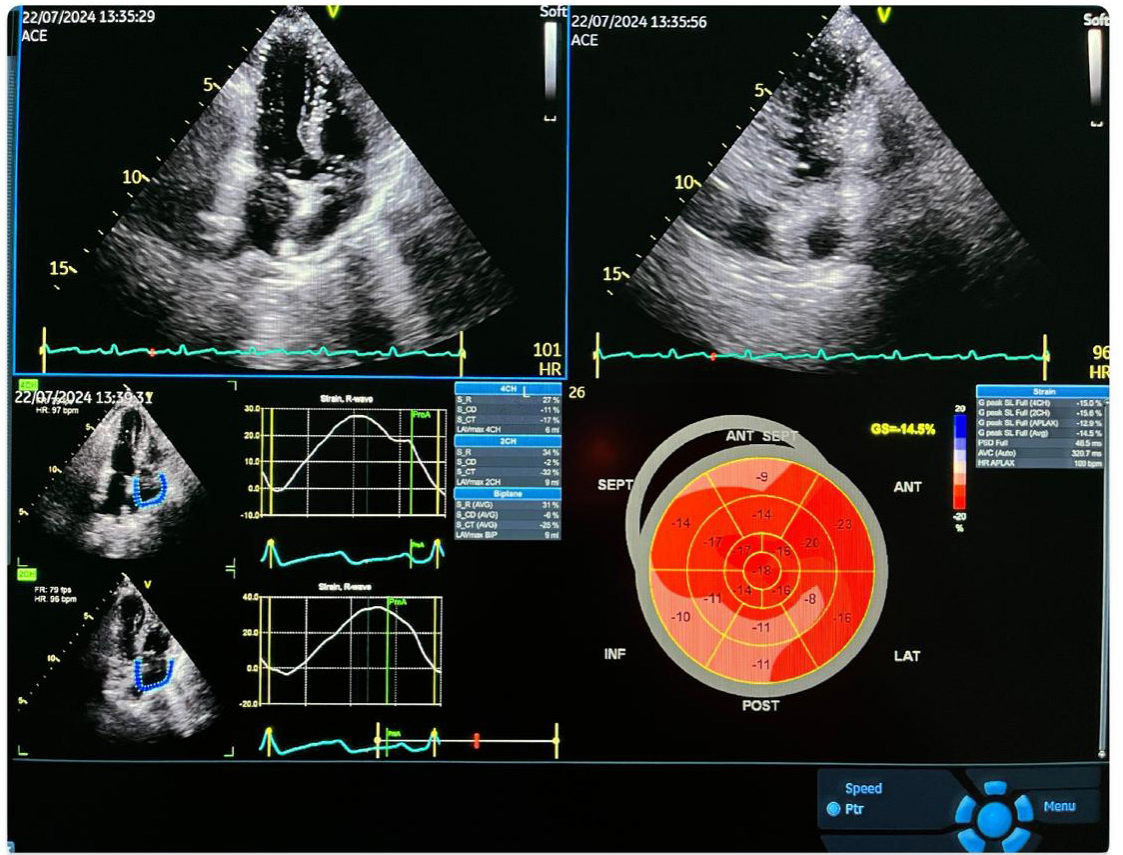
A typical echocardiographic and global longitudinal strain
